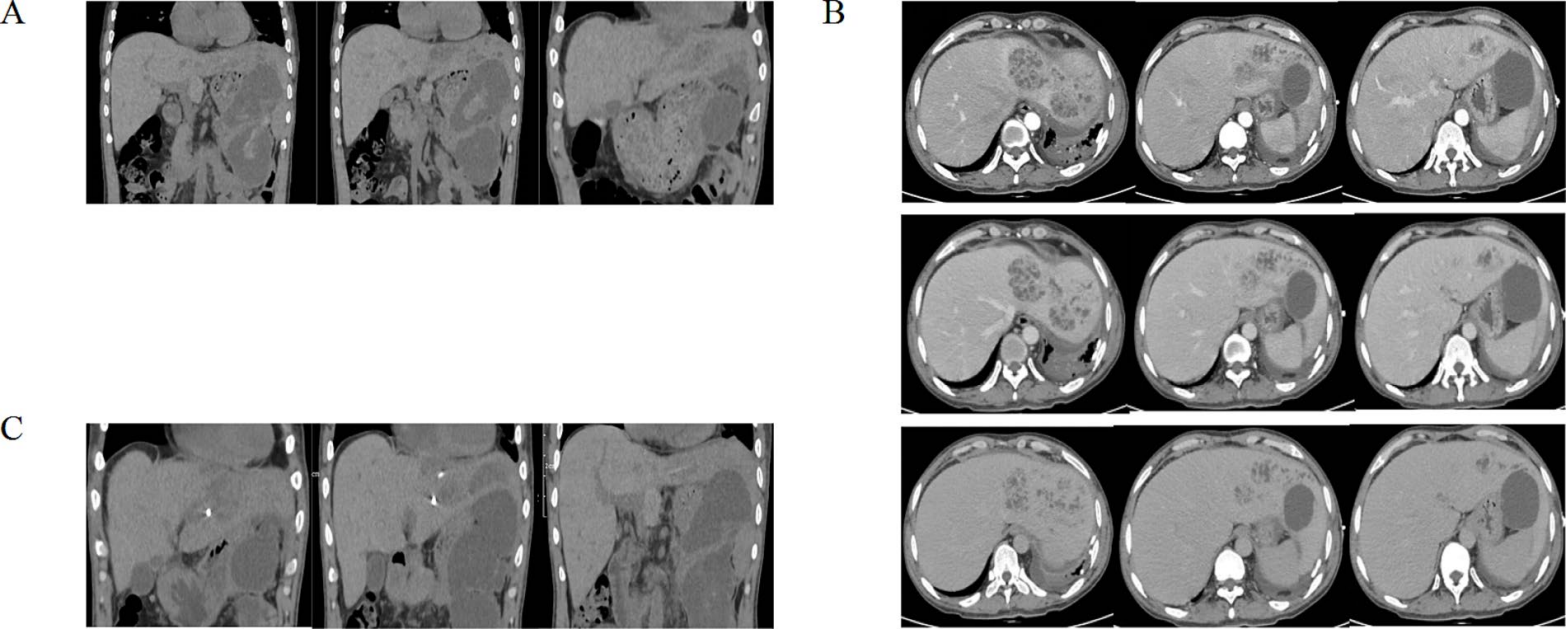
(A) Pre-admission whole abdominal CT revealed multiple space-occupying lesions in left hepatic lobe, mixed-density nodule in right subhepatic space, large space-occupying lesion in pancreatic tail and gallbladder stones. (B) Post-admission contrast-enhanced abdominal CT showed multiple abnormally enhancing cystic lesions in left hepatic lobe, consistent with infectious pathology, likely abscess formation; large pancreatic tail mass, likely benign, possibly pseudocyst formation with minor hemorrhage. (C) Follow-up CT after ultrasound-guided percutaneous catheter drainage of left hepatic abscess suggested pancreatic tail cyst with hemorrhage, and hepatic abscess suspected to communicate with pancreatic tail cyst or containing pancreatic secretions.
